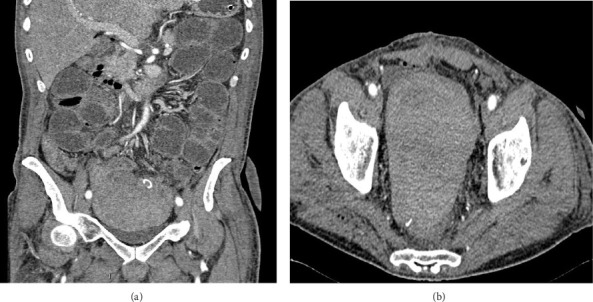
Computed tomography of the abdomen showing retroperitoneal hematoma (a) and axial view (b).
